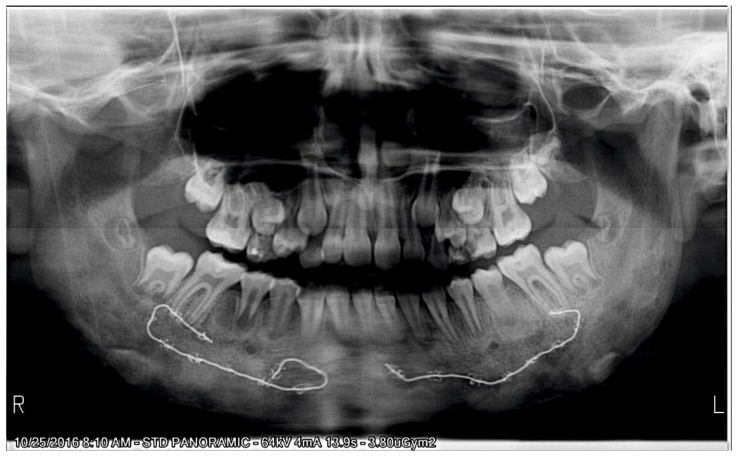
Post-splint therapy evaluation—panoramic radiograph.
